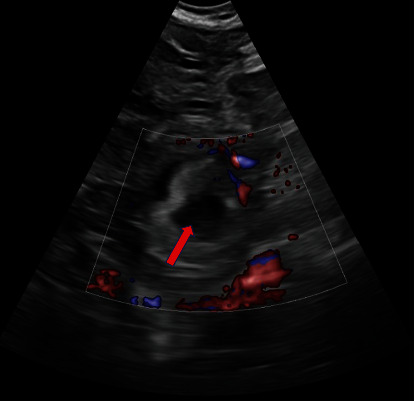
Longitudinal transvaginal ultrasound of the right adnexa demonstrates a thick walled hypoechoic cystic structure (red arrow) with weak peripheral vascularity (“ring of fire”). There is no fetal pole present.
